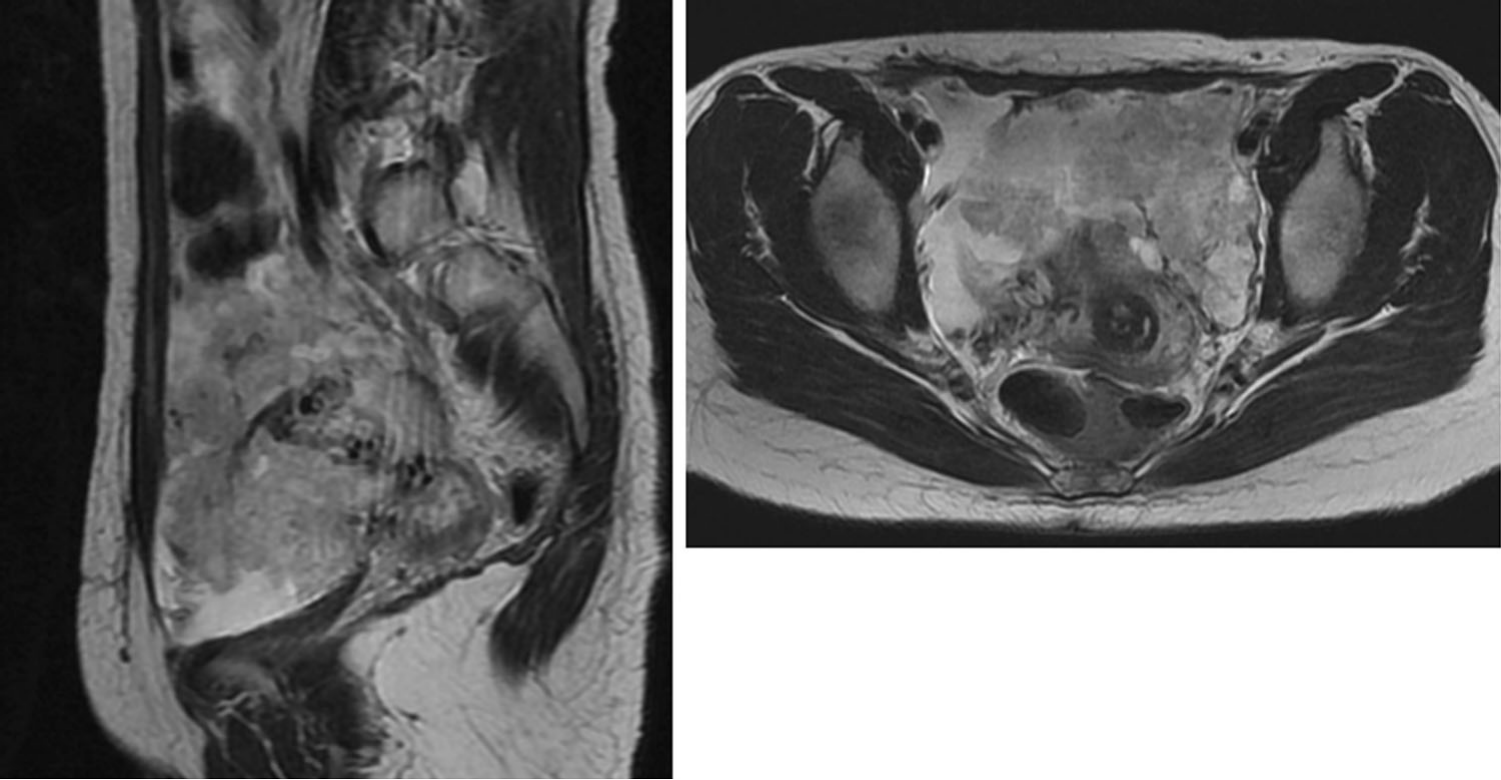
Pelvic MRI: T2-weighted imaging shows irregular shaped mass with *solid* components occupying the whole pelvis
